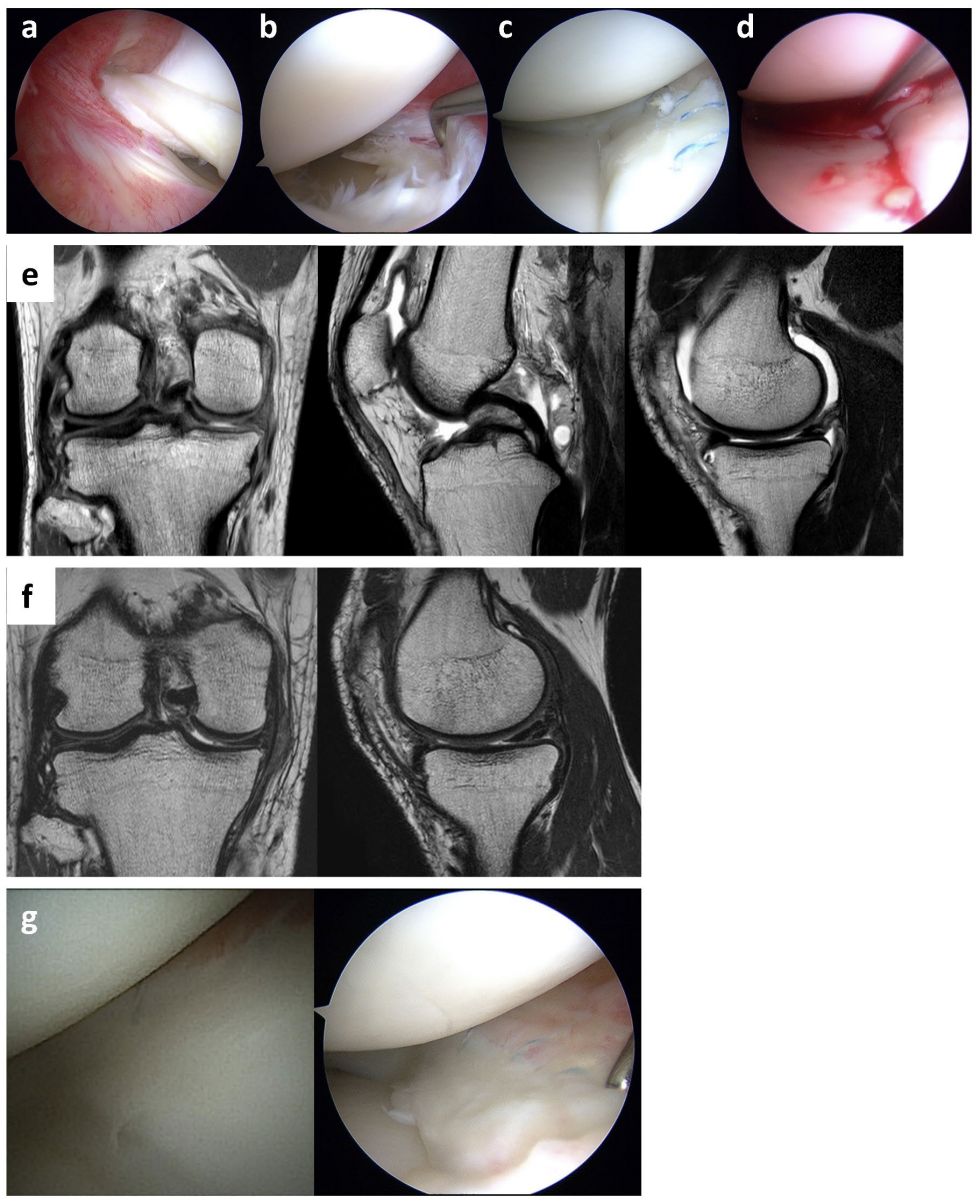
Treatment course of patient #8. (a) The right medial meniscus with bucket-handle tear displacement into the intercondylar region. (b) Arthroscopic confirmation of the tear in the avascular zone. (c) Inside-out sutures used for repair, followed by (d) P47K-WAS-MR gel application. (e) Preoperative T2-weighted MRI shows medial meniscus displacement in the coronal view and a double PCL sign in the sagittal view. (f) MRI at 3 months post-surgery indicates reduced meniscus position, graded as 2 on T2-weighted imaging. (g) Second-look arthroscopy reveals complete healing with views from the Nanoscope^®^ (left) and conventional arthroscope (right).
